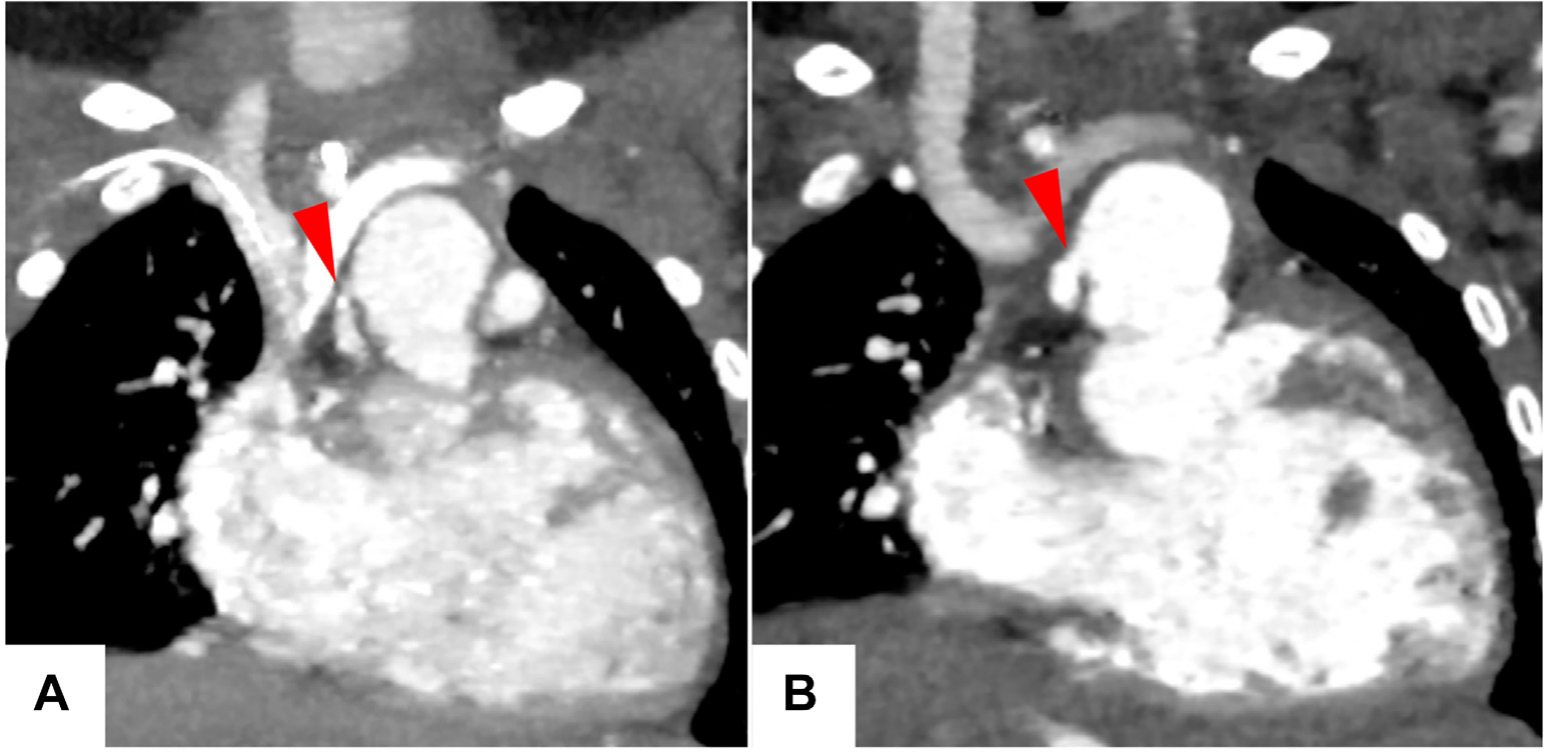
(A) Preoperative computed tomography image showing stenosis (arrowhead) at the anastomosis between native ascending aorta and neoaorta. (B) Postoperative computed tomography image showing its resolution (arrowhead).
